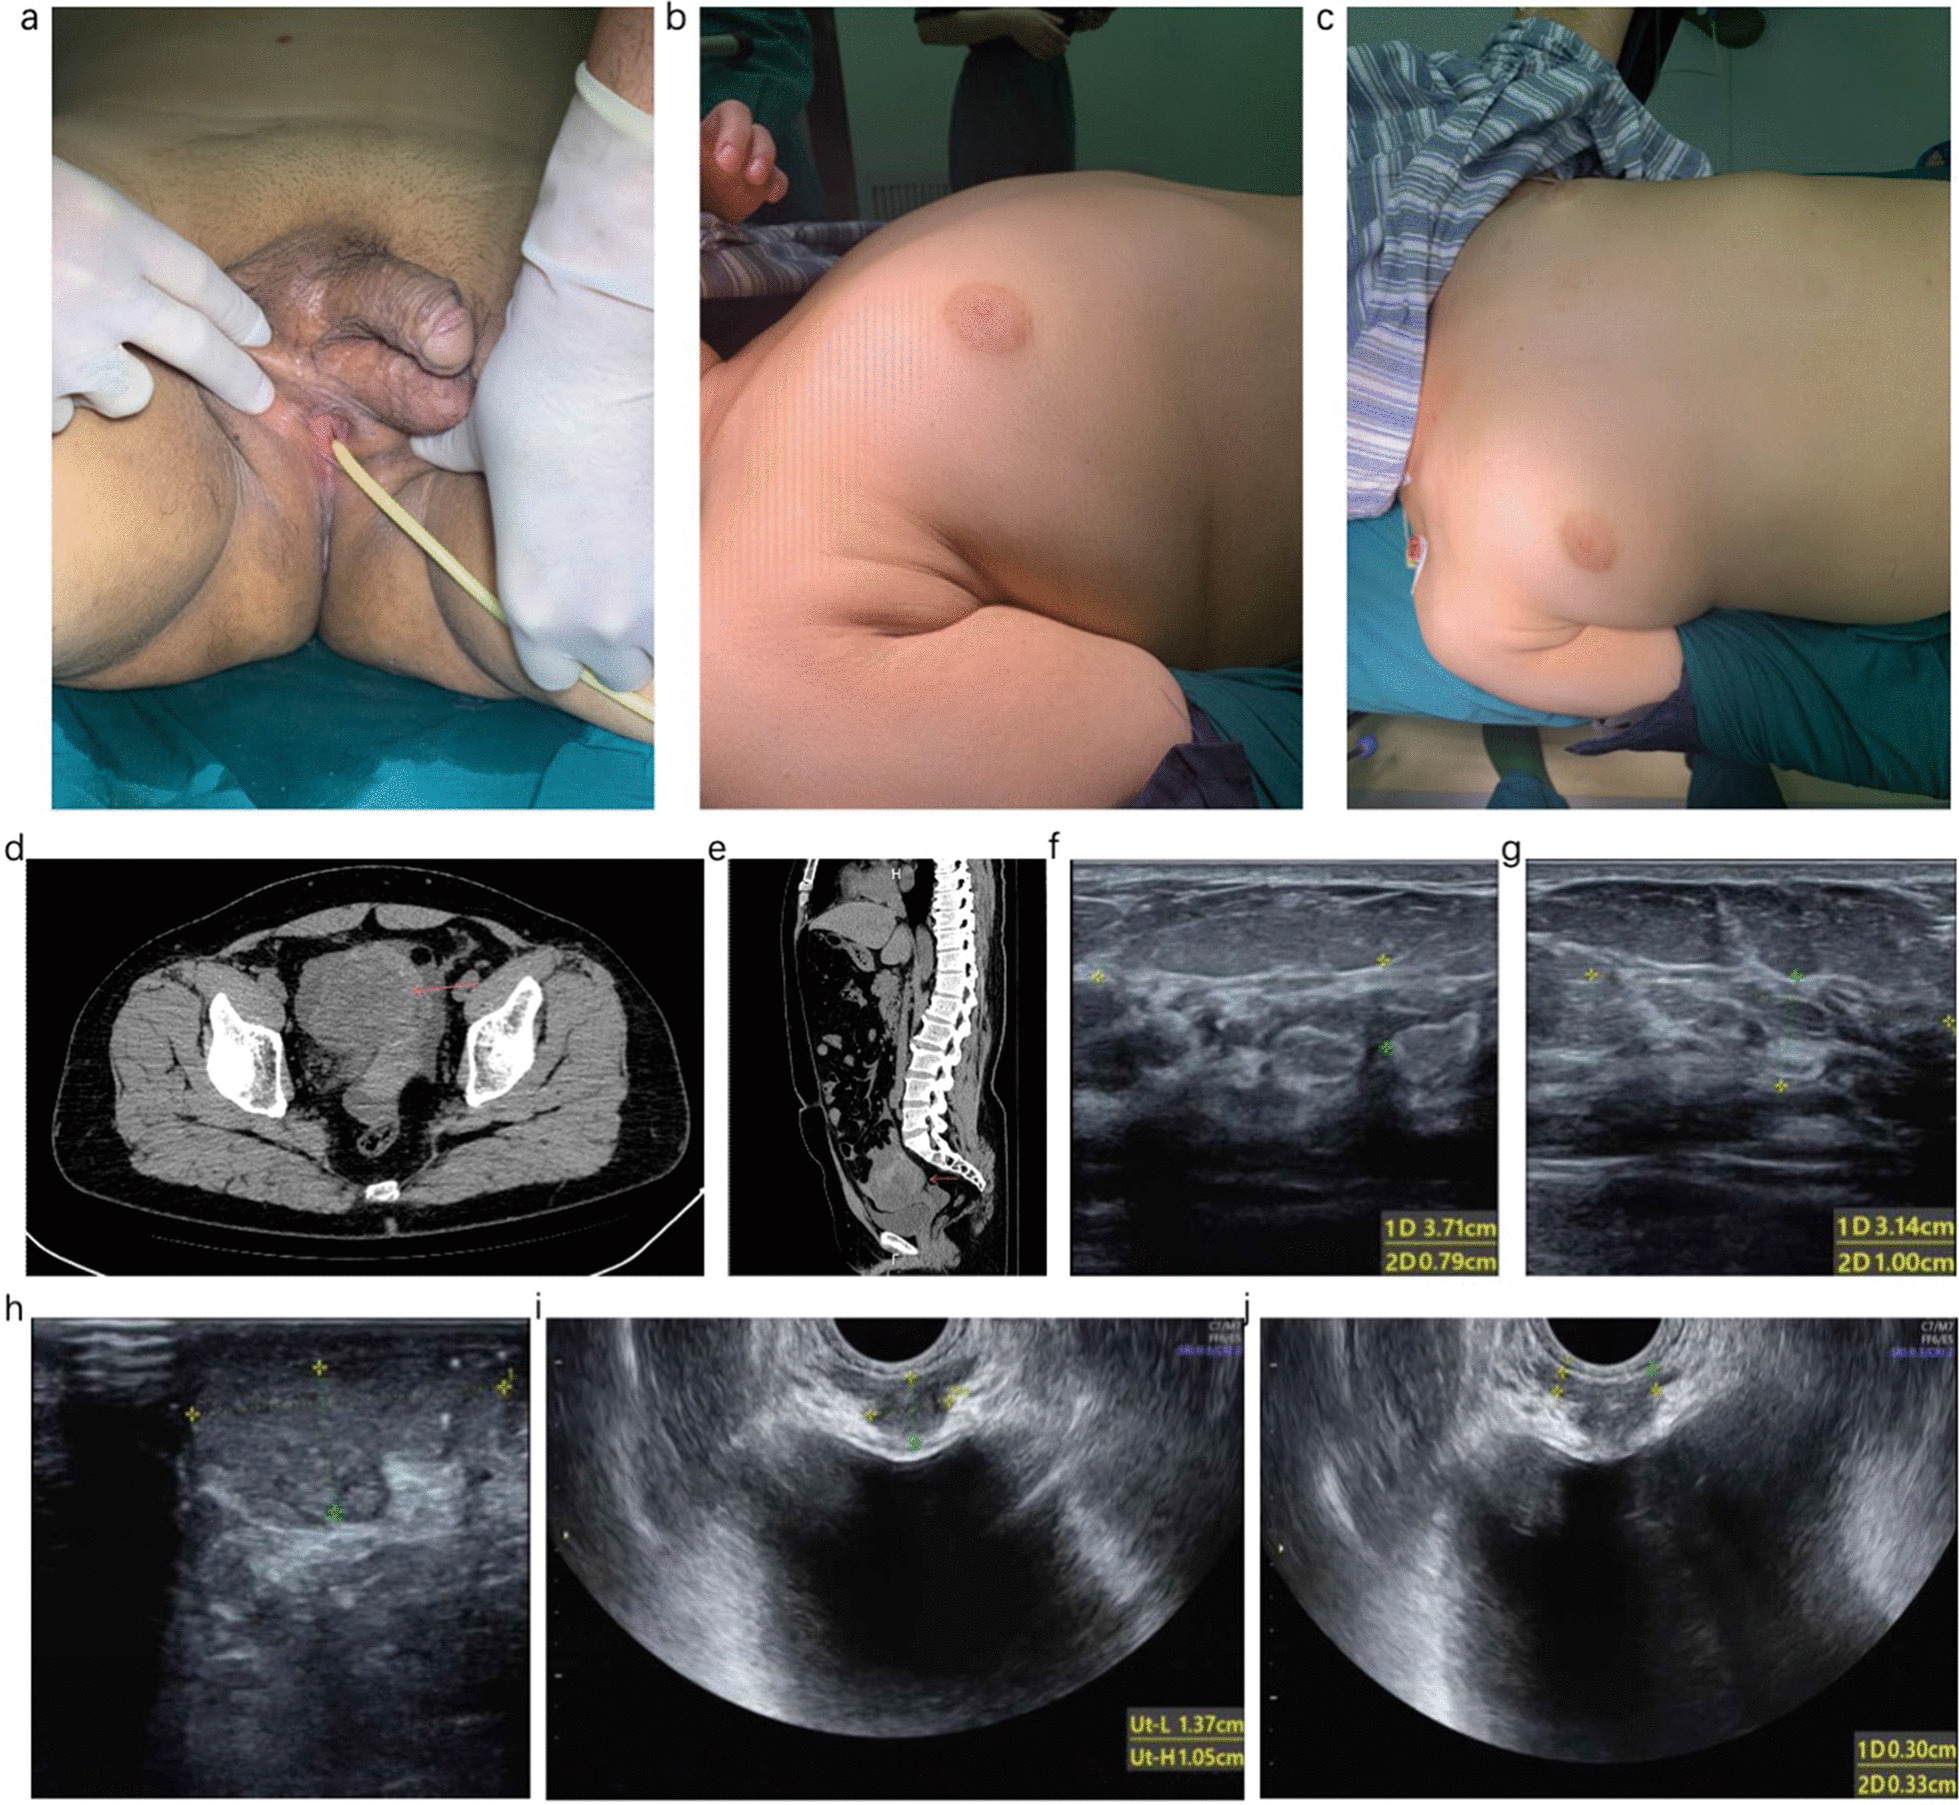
a Ambiguity of external genitalia with normal size penis and female-like urethral opening in the perineum area. b and c Gynecomastia (Grade II, Rohirich Classification) [5]. d The transverse plane of CT of the pelvis revealed a tumor located in the right pelvis (red arrow). e The sagittal plane of CT revealed a tumor (red arrow) located between the bladder and rectum. f and g Ultrasound of bilateral mammary gland. h Ultrasound of the left testicle. i Ultrasound of prostate-like structure in patient’s prostate area. j Ultrasound of bilateral seminal vesicle gland
